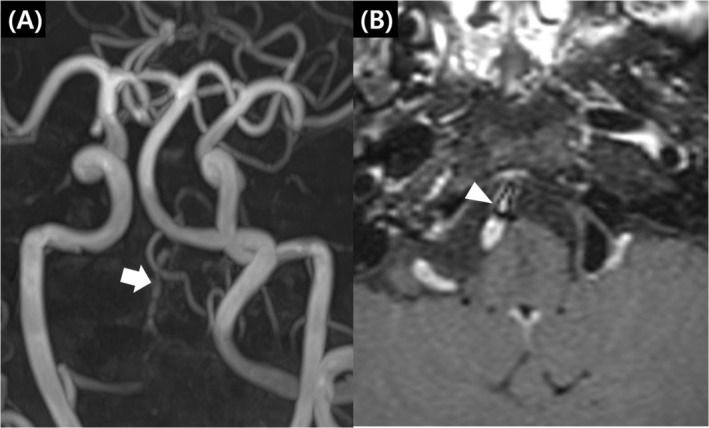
Pretreatment imaging of the acute, unruptured right V4 vertebral artery dissection (January 7, 2025). (A) Frontal maximum intensity projection (MIP) MR angiography (MRA) showing focal narrowing and luminal irregularity of the right V4 segment (arrow). (B) Axial T1‐weighted high‐resolution vessel wall MRI showing a hyperintense intramural hematoma and intimal flap causing eccentric luminal narrowing (arrowhead).
